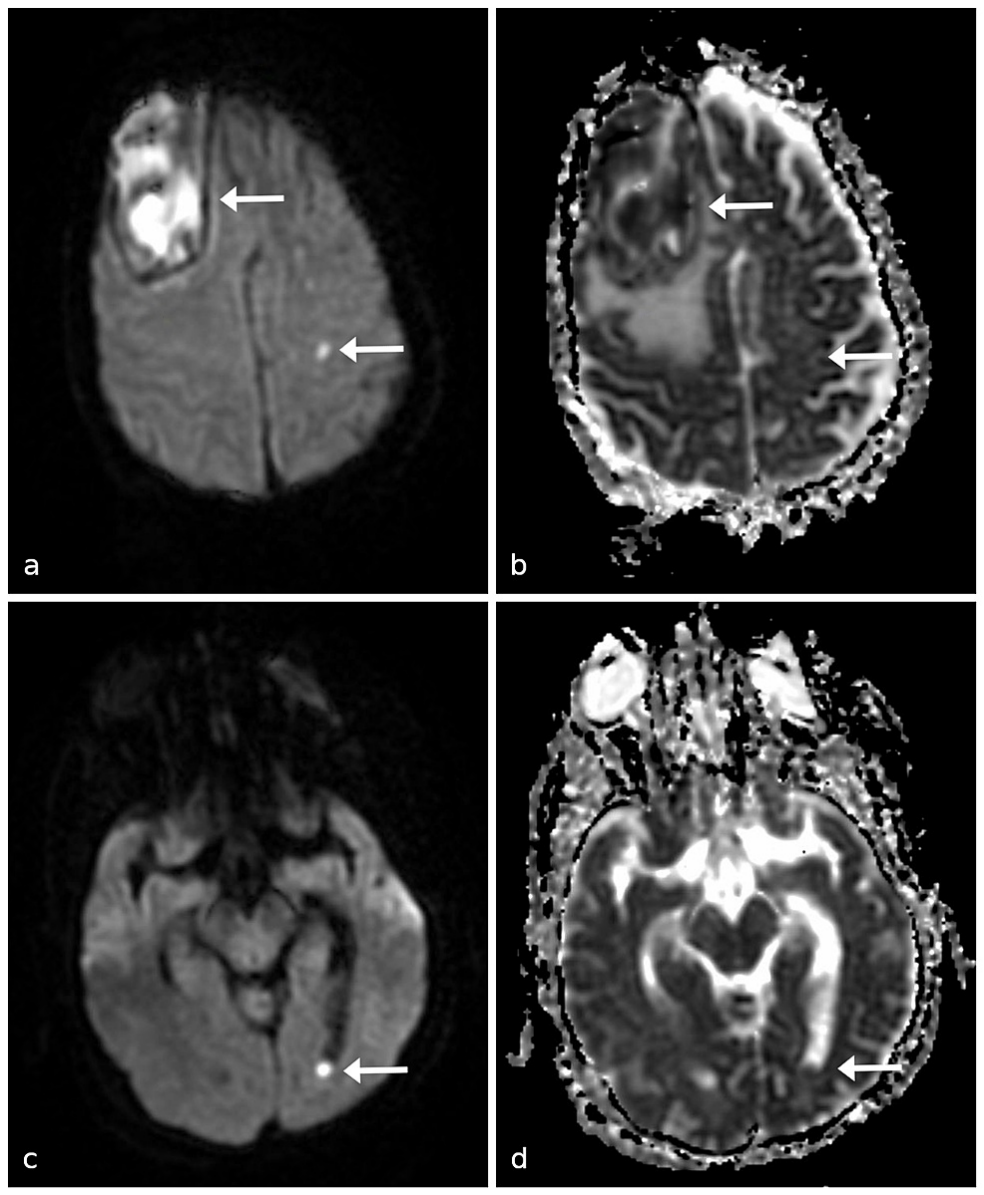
(a) MRI DWI and (b) corresponding ADC map showing restricted diffusion within the right frontal lesion; (c) MRI DWI and (d) corresponding ADC map showing restricted diffusion in the dependent lateral ventricle. MRI, magnetic resonance imaging; DWI, diffusion-weighted imaging; ADC, apparent diffusion coefficient
